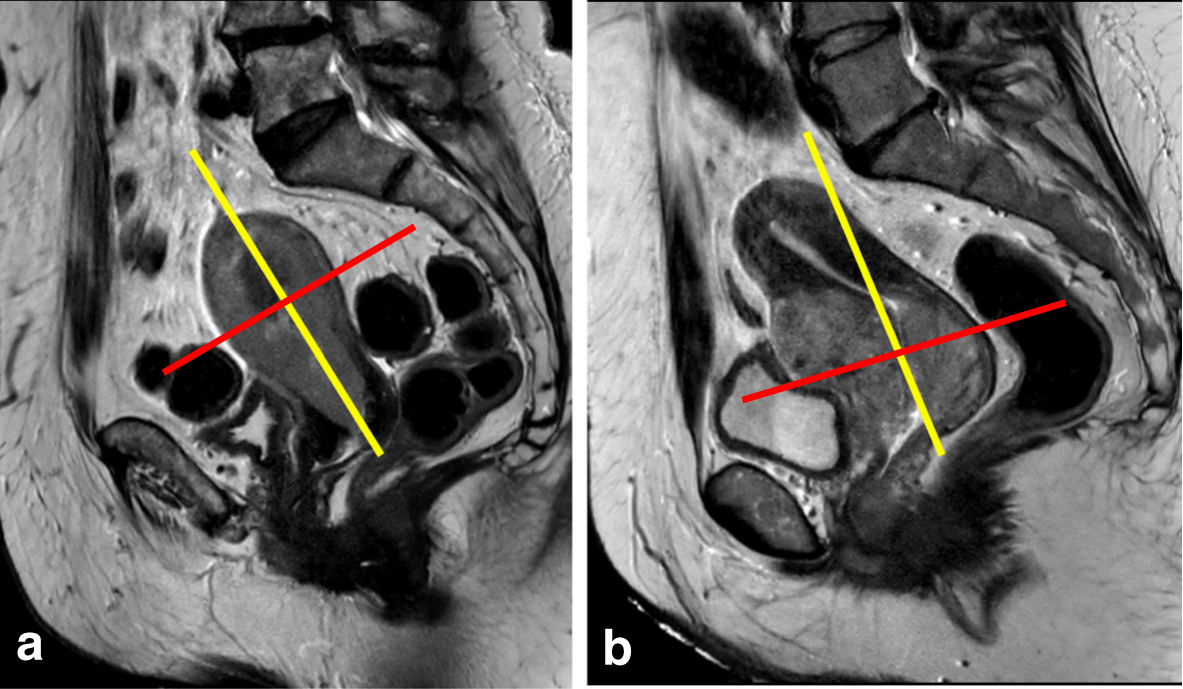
Planning of MRI sequences in endometrial (a) and cervical (b) cancers. Yellow line indicates the coronal plane, red line indicates the axial oblique plane
